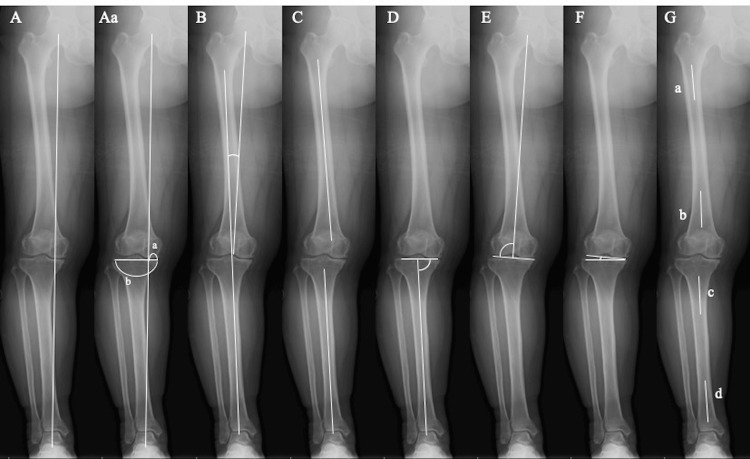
X-ray measurement parameters. X-ray evaluation was performed using standing anteroposterior one-leg standing full-length radiographs of the lower limb. (A) Mechanical axis (Aa) The % MA is calculated as the ratio of the horizontal distance from the medial edge of the tibial plateau to the intersection of the MA. % MA = a/b. (B) HKA: Angle between the femoral and tibial functional axes. (C) FTA: Angle between the femoral and tibial anatomical axes. (D) MPTA: Angle between the tibial anatomical axis and the joint line of the proximal tibia. (E) LDFA: Angle between the femoral functional axis and the distal femoral joint line. (F) JLCA: Angle between the distal femoral and proximal tibial joint line. (G) FBA: Angle between the proximal 1/4 line through the center of the femoral diaphysis and the distal 1/4 line. TBA: Angle between the proximal 1/3 line through the center of the tibial diaphysis and the distal 1/3 line.
